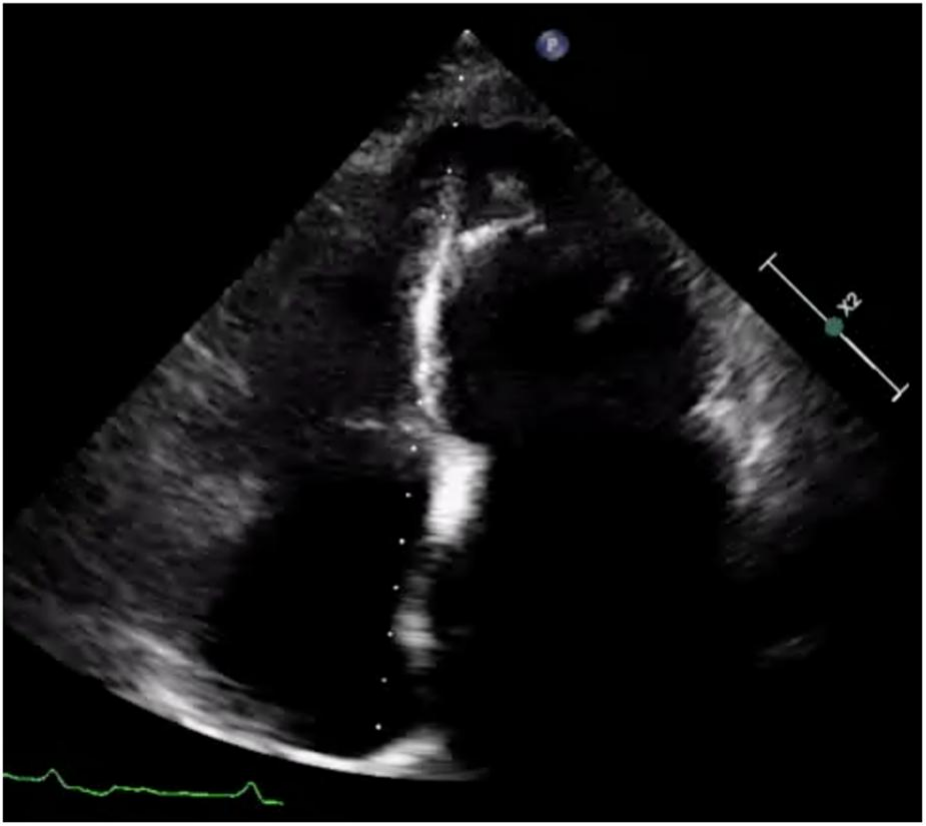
Apical 4-chamber view of the left ventricle showing apical hypertrophy in addition to asynergy in the apical walls with high echogenicity.
